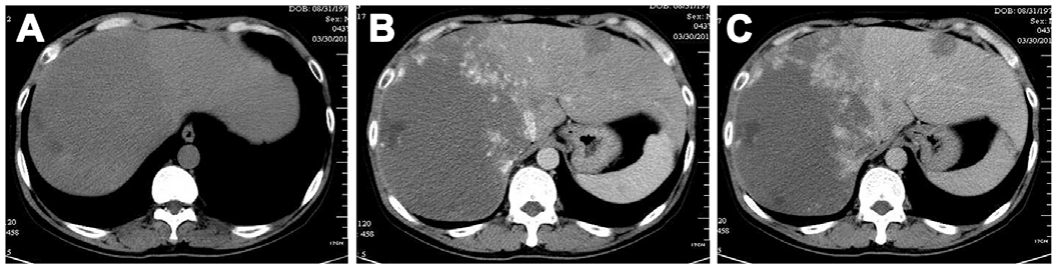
CT plain scan. An oval, low-density lesion with clear boundaries and central liquefactive necrosis (A). CT enhanced scan showing nodular enhancement at the edges of the lesion during the early arterial phase, and a higher density than the normal liver (B and C), appearing as the “fast out, slow in” sign.
